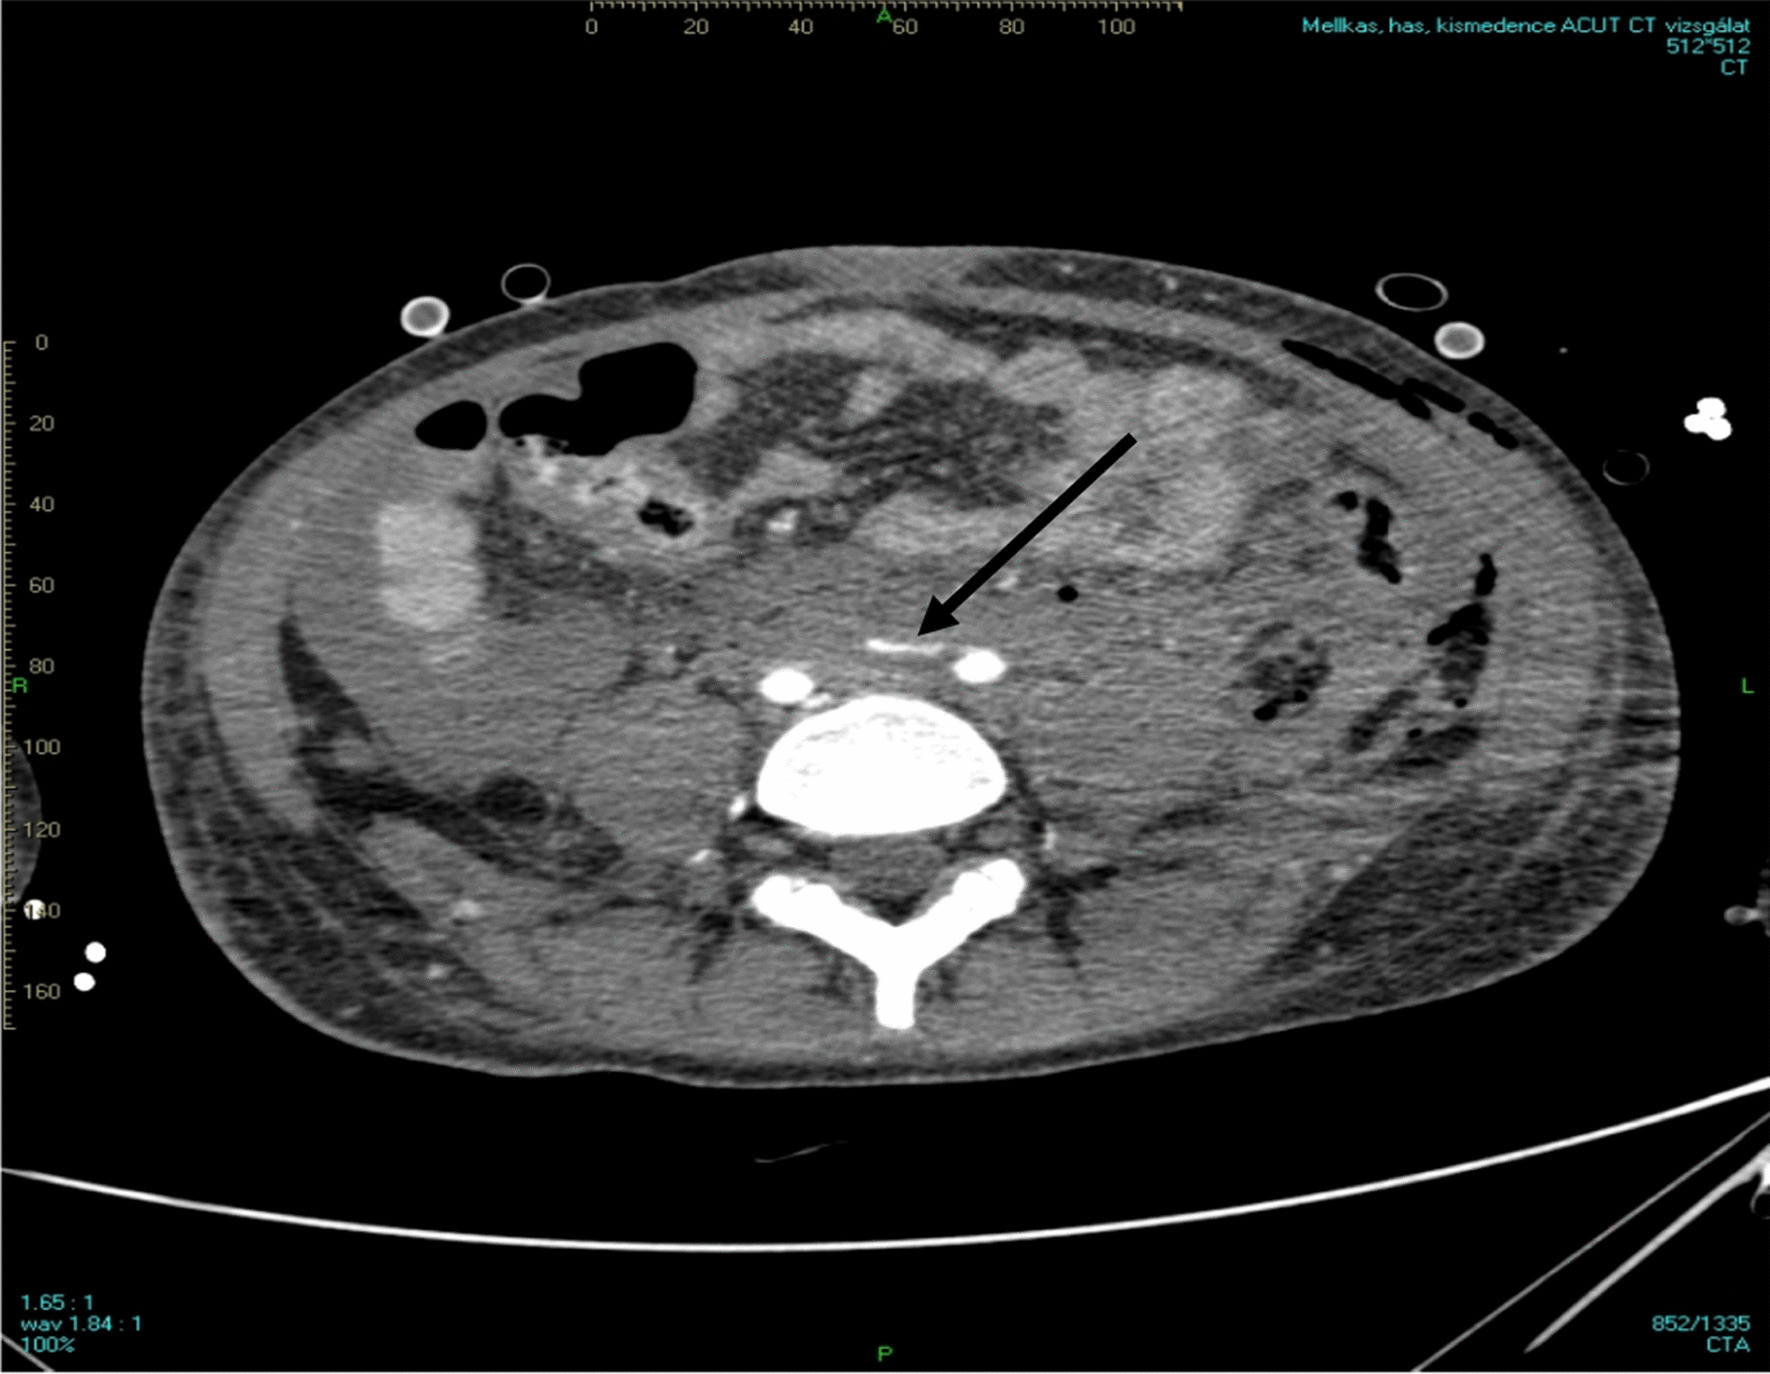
The computed tomography shows that the left-sided arteria iliaca had a radiocontrast extravasation due to artery rupture (arrow)
